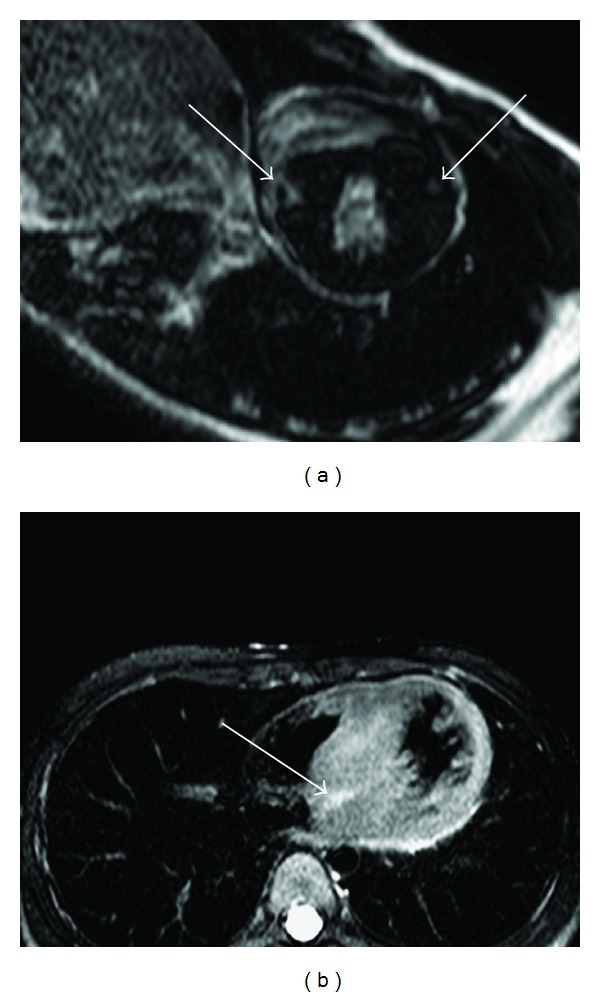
77-year-old female with hypertrophic cardiomyopathy presenting with chest pain. Delayed-enhancement MRI (a) shows patchy enhancement at the anterior and inferior septal myocardium (arrows). T2-weighted cardiac MRI (b) shows myocardial edema at the inferior and inferior septal myocardium (arrow).
